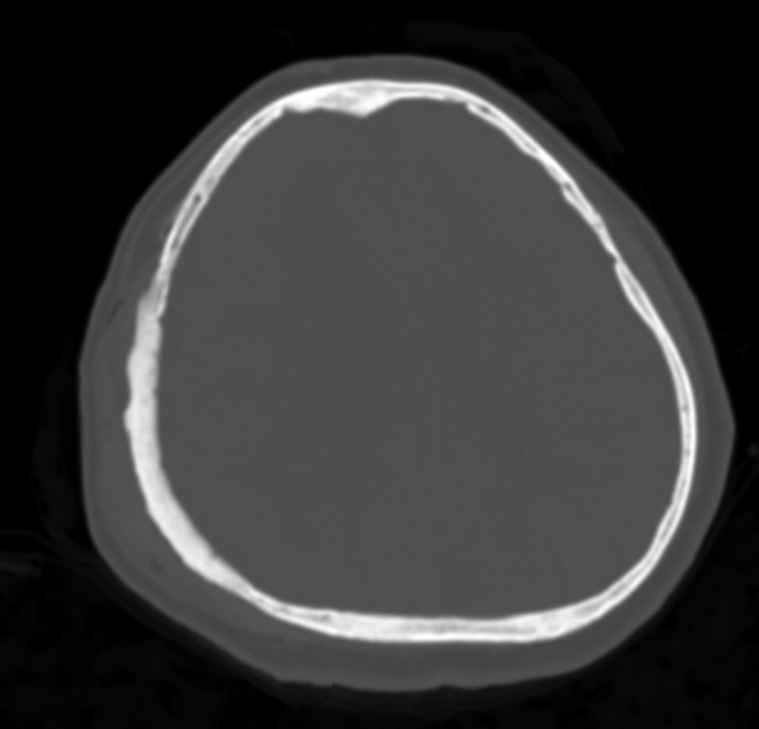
Follow-up cranial computed tomography obtained 1 year after surgery. Axial bone-window image showing no evidence of recurrence or regrowth.
